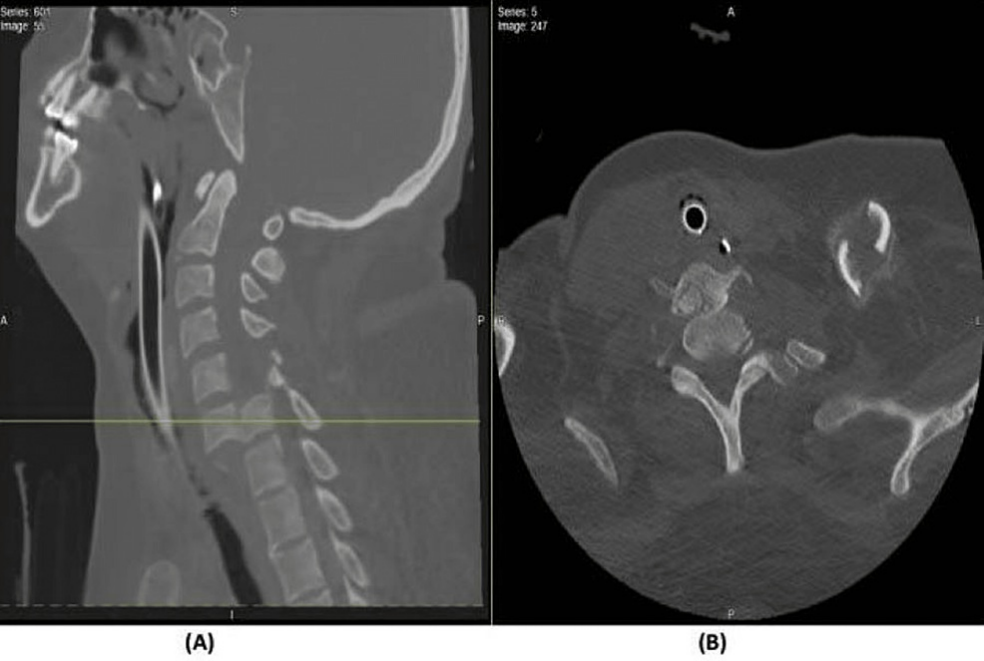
CT scan of C6-7 fracture dislocation (A) Cervical spine CT scan sagittal cuts demonstrating a total fracture dislocation of C6 over C7; (B) cervical spine CT scan axial cuts demonstrating the corresponding level of C6–C7.
